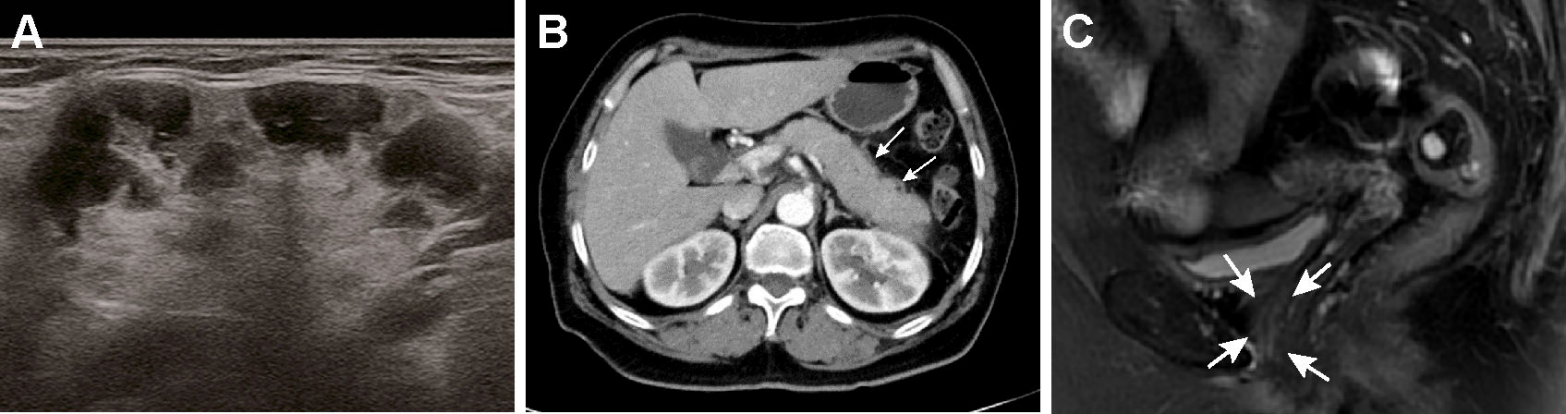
Radiological manifestations of the salivary glands and pancreas and significant improvement of periurethral mass after treatment. (A) An ultrasound image of the right submandibular gland showing numerous patchy hypoechoic regions inside the glandular parenchyma. (B) Contrast-enhanced CT scan of the abdomen showed the pancreas appears diffusely enlarged with a hypodense peripancreatic rim (white arrows). (C) After 3 months of treatment, MRI of the pelvis revealed marked decrease in size of the periurethral mass (white arrows).
